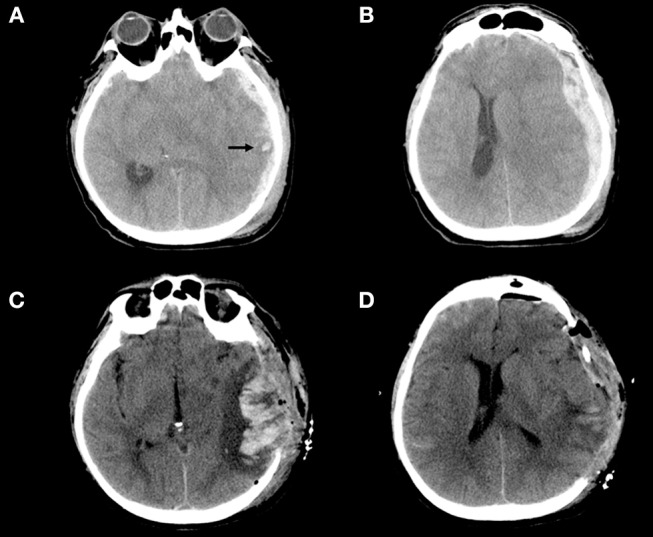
(A–D) Example of blossoming contusion in a 52-year-old male patient treated with DC for traumatic acute subdural hematoma. The patient sustained a close head injury in a motor vehicle accident and 2 h after trauma was admitted at our hospital with a Glasgow Coma Scale score of 6 (E1V1M4) and was put under mechanical ventilation. The pupils were bilaterally equal and reactive. (A,B) (Pre-operative CT scan). Pre-operative CT scan, immediately performed after admission of the patient described above, showing a large left acute subdural hematoma with a small temporal contusion [black arrow–(A)] and midline shift (B); then the patient was immediately operated. (C,D) (Post-operative CT scan) Post-operative CT scan performed about 3 h after DC demonstrating massive contusion expansion (C) after wide DC (D). During surgery bone flap was not replaced for massive brain swelling without evidence of bleeding/contusion enlargement at the level of temporal lobe.
